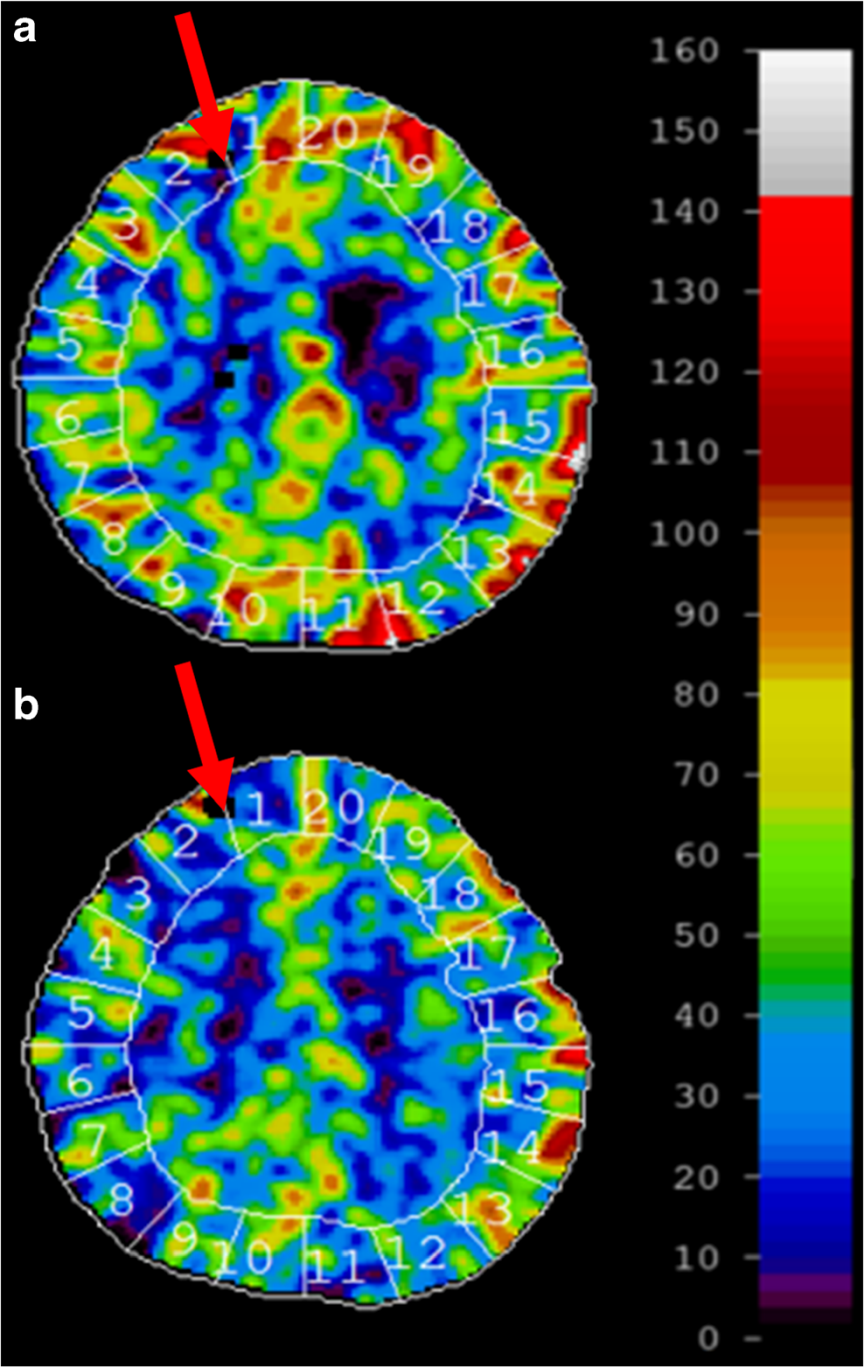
Scans from a Xe-CT (a) day 1 and (b) day 3. The color bar shows blood flow values in ml/100 g/min; the arrows point at the position of the optical probe tip. The blood flow in the region neighboring the optical probe was approximately 11 and 27 ml/100 g/min at day 1 and day 3, respectively
